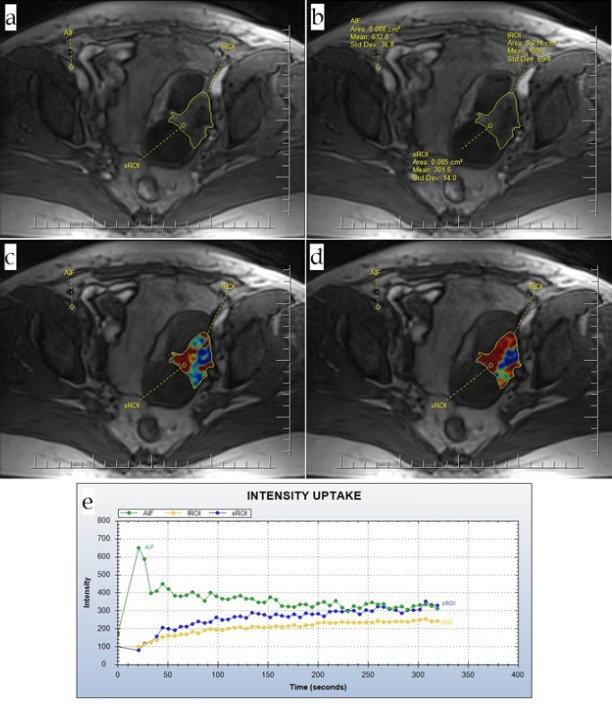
Type II Intensity Curve Along with the Corresponding Quantitative Measurements on the Patient’s MRI; (a) Source; (b) Details of the source; (c) Kep; (d) Ktrans; (e) TIC
